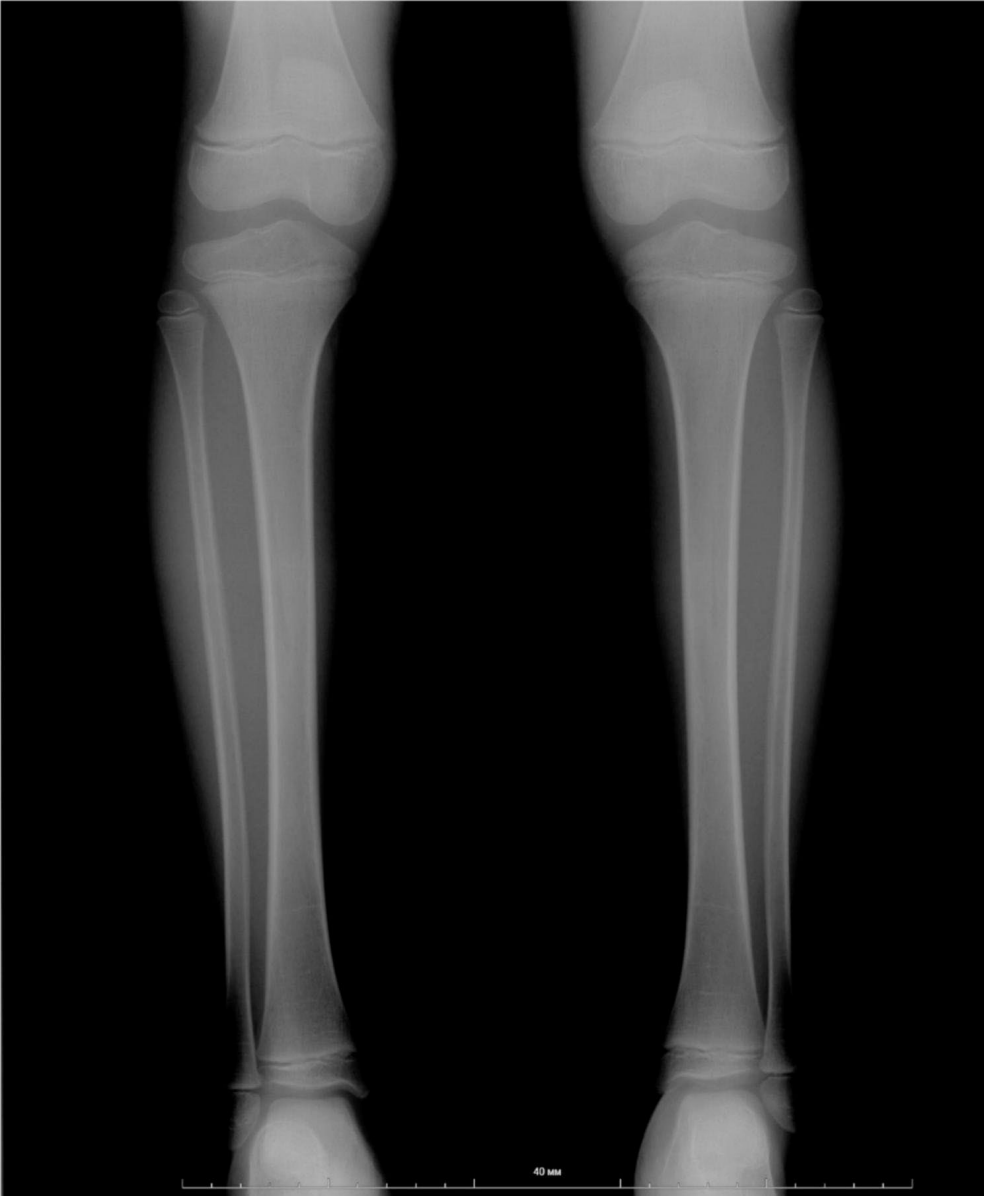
The radiograph of the legs of the probands sister (7 years old) in a direct projection: genu valgum and a mild decrease in bone density
